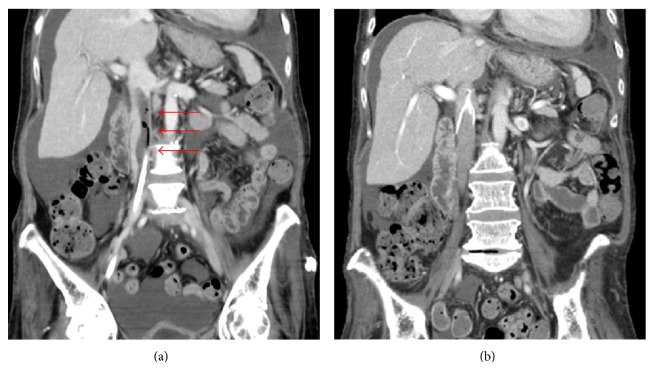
(a) Contrast-enhanced computed tomography (CT) demonstrated nonobstructive thrombus (*arrows*) with air density extending from the CVC via the right femoral vein to the suprarenal IVC, just above the renal vein confluence. (b) Follow-up CT established that there was no IVC thrombus or thrombus in the filter.
